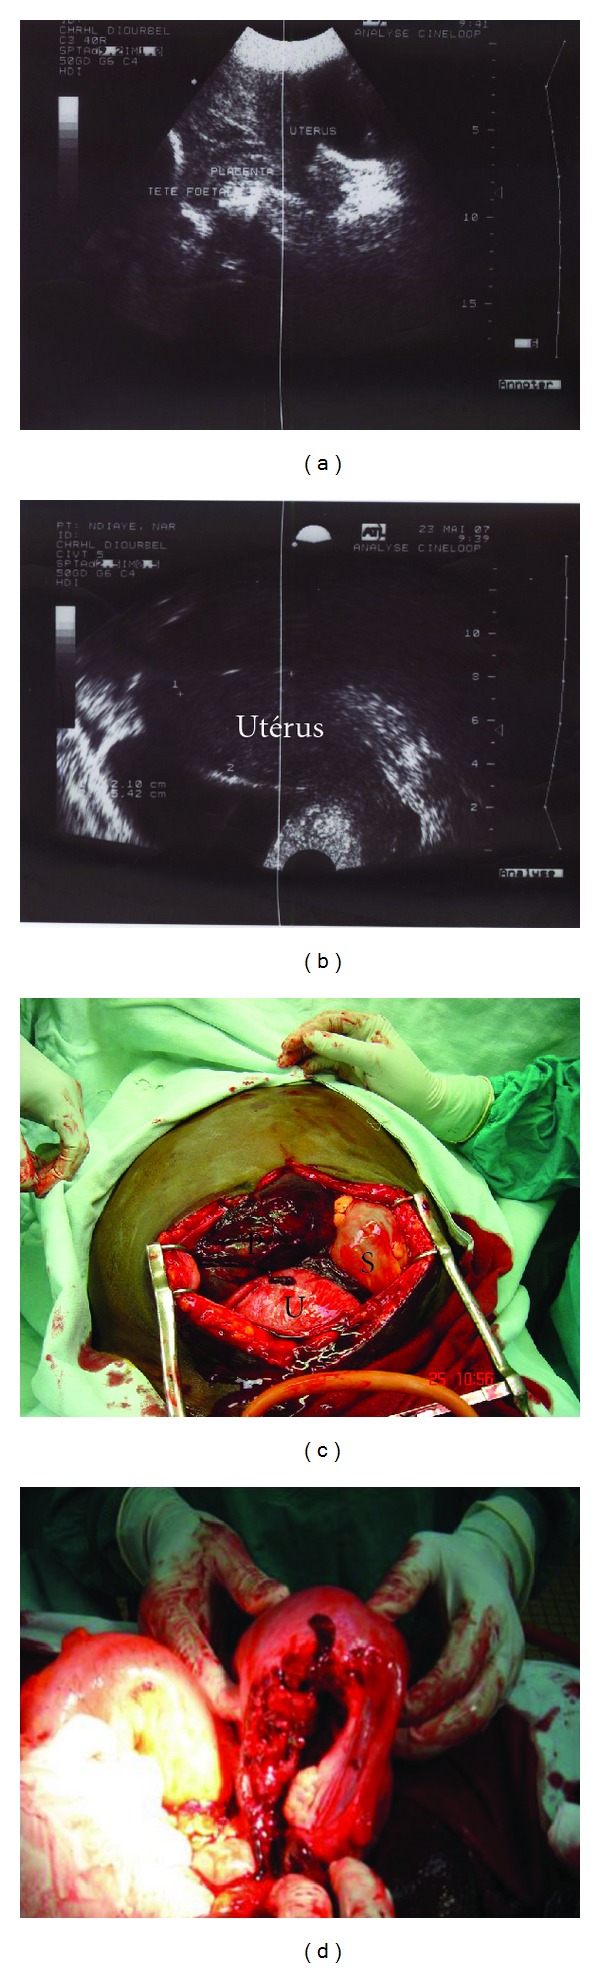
(a) Ultrasound image showing empty uterus, placenta, and fetal head; (b) empty uterus (extension); (c) visualization of the uterus (U), the gestational sac (S), and placenta (P) after opening the abdomen; (d) image showing uterine rupture.
